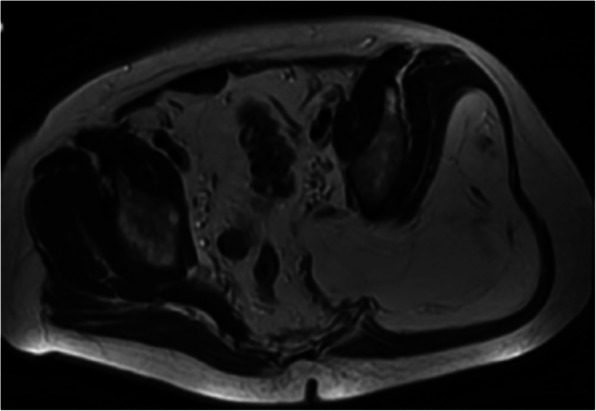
Patient 1: MRI of the liposarcoma within the sciatic notch
